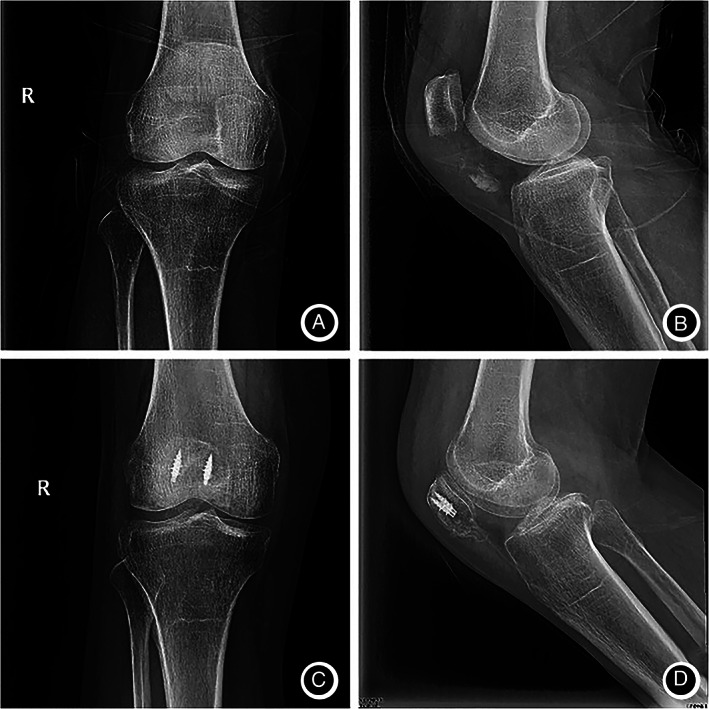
Anterioposterior and lateral knee radiographs of a 65‐year‐old female patient. (A,B) Patients with distal pole patellar fractures; (C,D) after treatment with anchor and Krackow‐“8” suture fixation.
